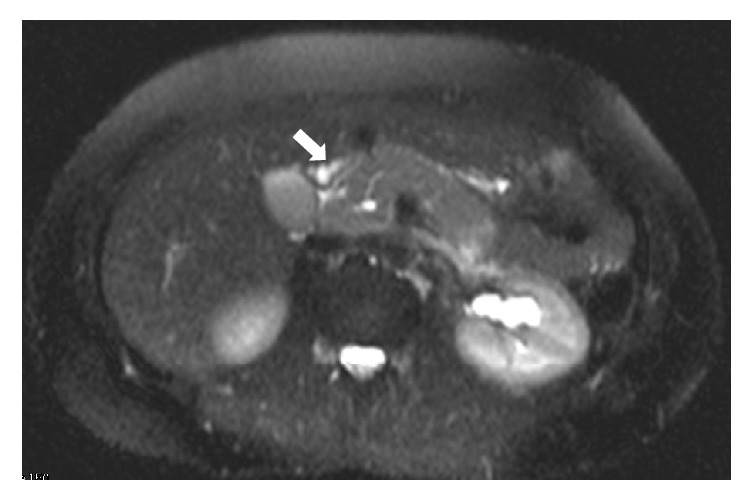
Magnetic resonance image of the abdomen with contrast (T2 sequence) shows heterogeneous signal pancreatic head mass measuring 4.5 cm, triggering concern for primary pancreatic carcinoma with associated pancreatic duct dilatation and peripancreatic lymphadenopathy (white arrow).
